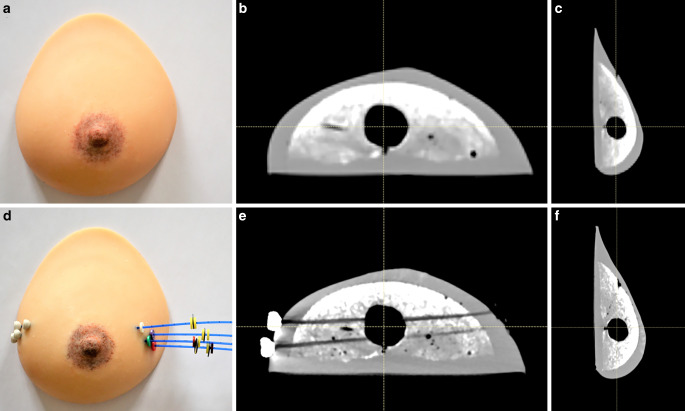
Photographic images (a) and CT scans of the breast model in axial (b) and sagittal view (c). The imitation of the “tumor bed” appears hypointense on CT. The catheter positions (d) within the breast model are visualized by CT scan (e–f)
